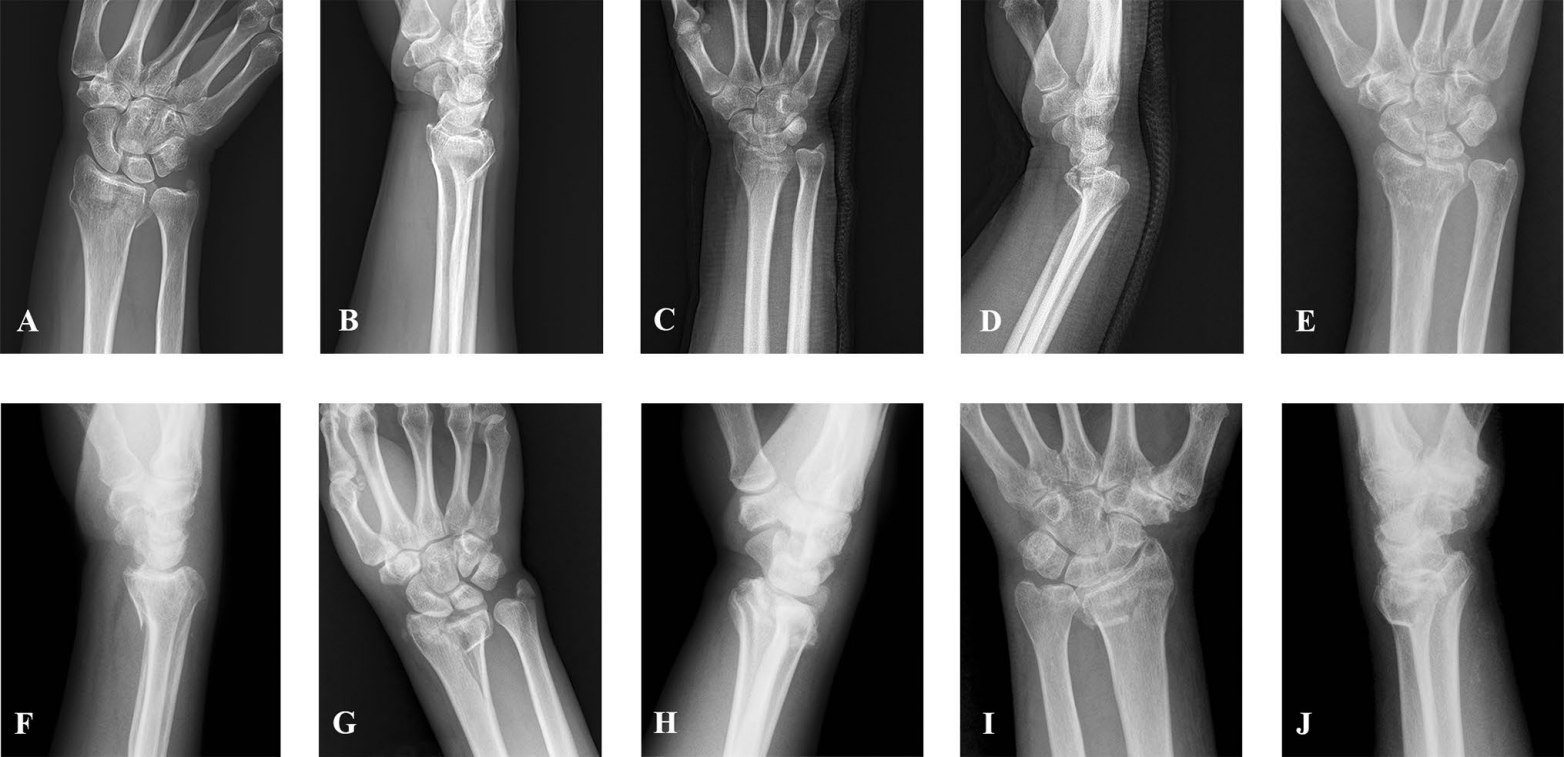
a, b show “┤” type fractures. c, d show “┤” type fractures. e, f show “┤” type fractures. g, h show “├” type fractures. i, j show “┼” type fractures. a, c, e, g, i the anteroposterior X-ray film; b, d, f, h, j represents the lateral X-ray film. Although fracture lines can be seen in (a, c), it is difficult to distinguish the distribution of fracture lines on the dorsal and dorsal sides. Although there are overlapping ulnar shadows in (b), dorsal displacement of the fracture block can be inferred. Similarly, the bone mass in (d) has a dorsal displacement. The sagittal fracture line can be clearly seen in (e, g) and the position of the fracture block can also be determined. However, it is difficult to obtain effective information in figure (f, h). The radius height shortening and fracture line distribution were observed in (i). Separation and displacement of the volar and dorsal fractures of the distal radius can be observed in (J)
